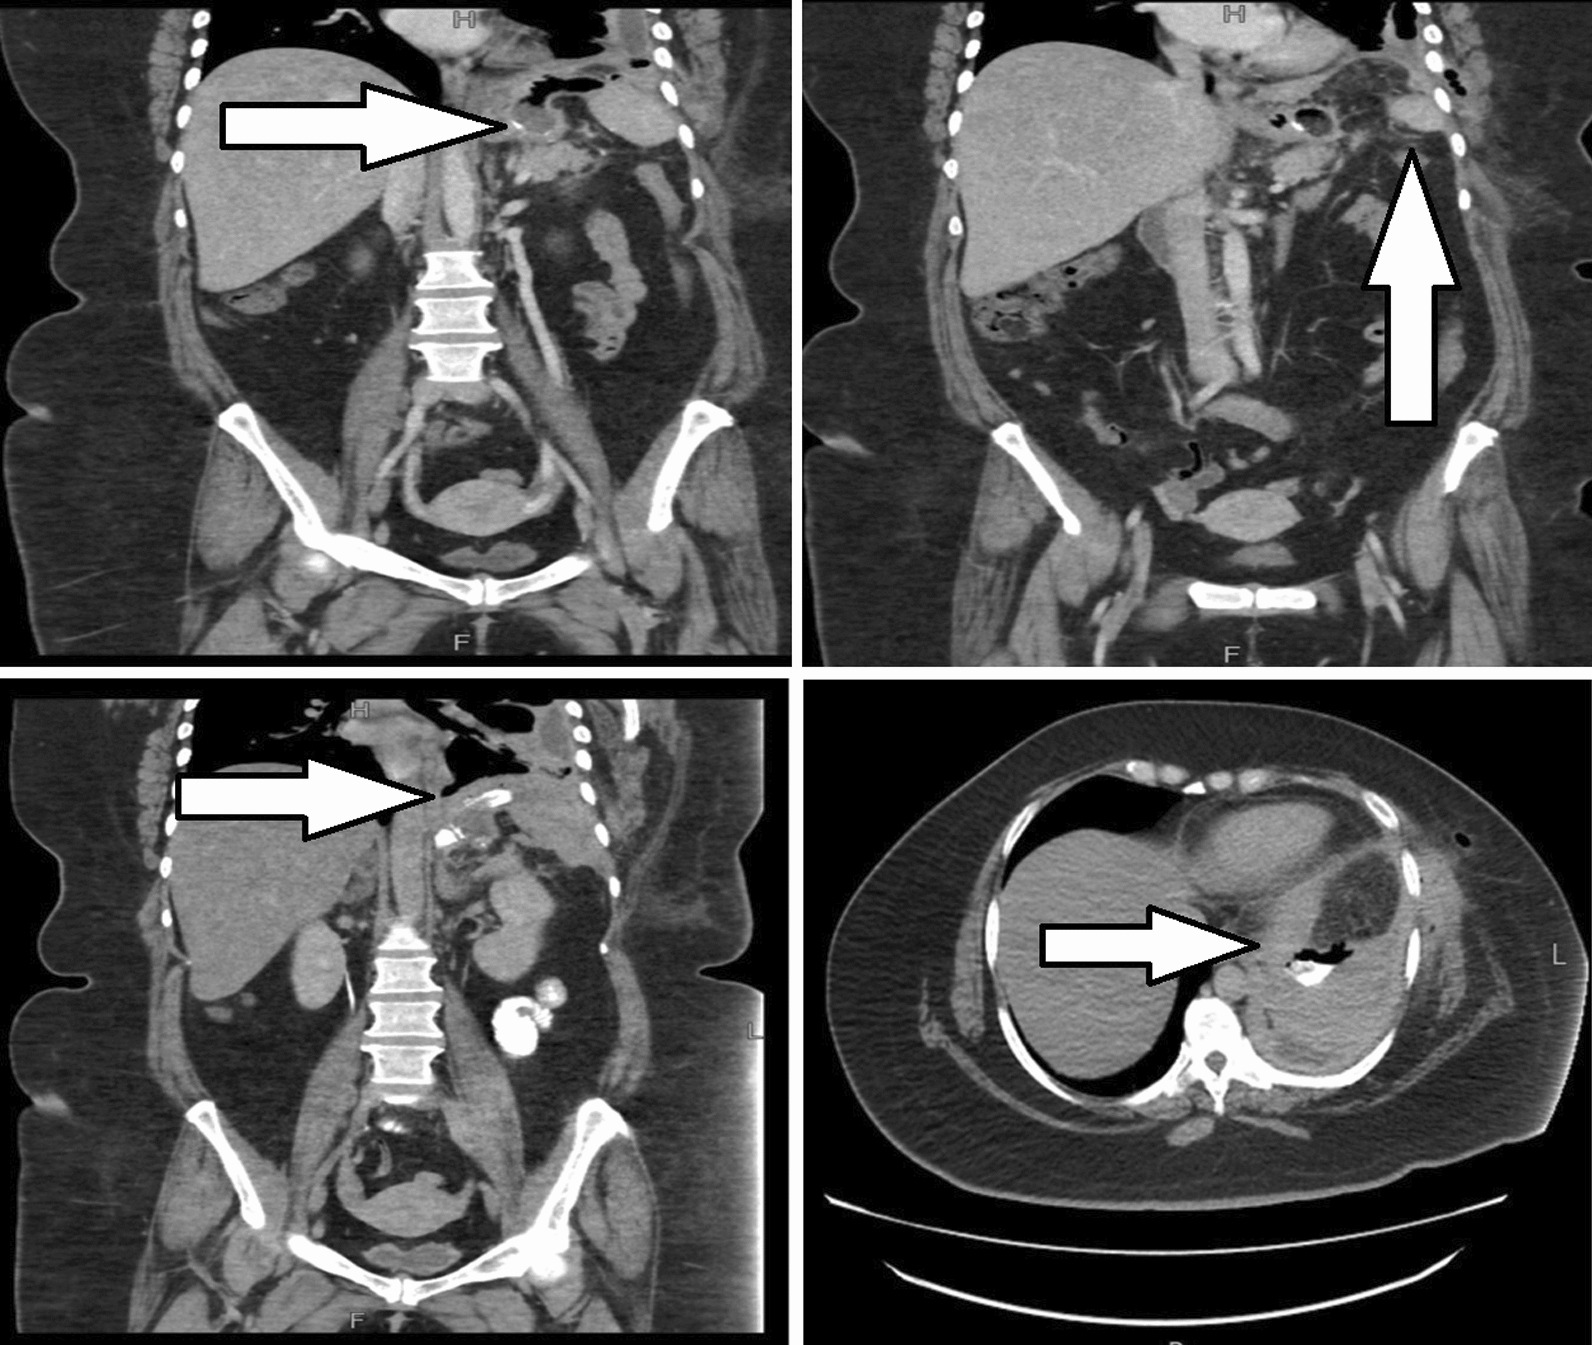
A–D CT scan of abdomen and pelvis performed with IV and oral contrast demonstrated (A, B) abnormal communication between gastric fundus, left pleural reflection over left hemi diaphragm as well as the left chest wall, representing gastro-pleural and gastro-cutaneous fistulae (arrows). This is confirmed by the administration of oral contrast (C, D). With contrast tricking from the gastric fundus into the left pleural cavity (arrows)
